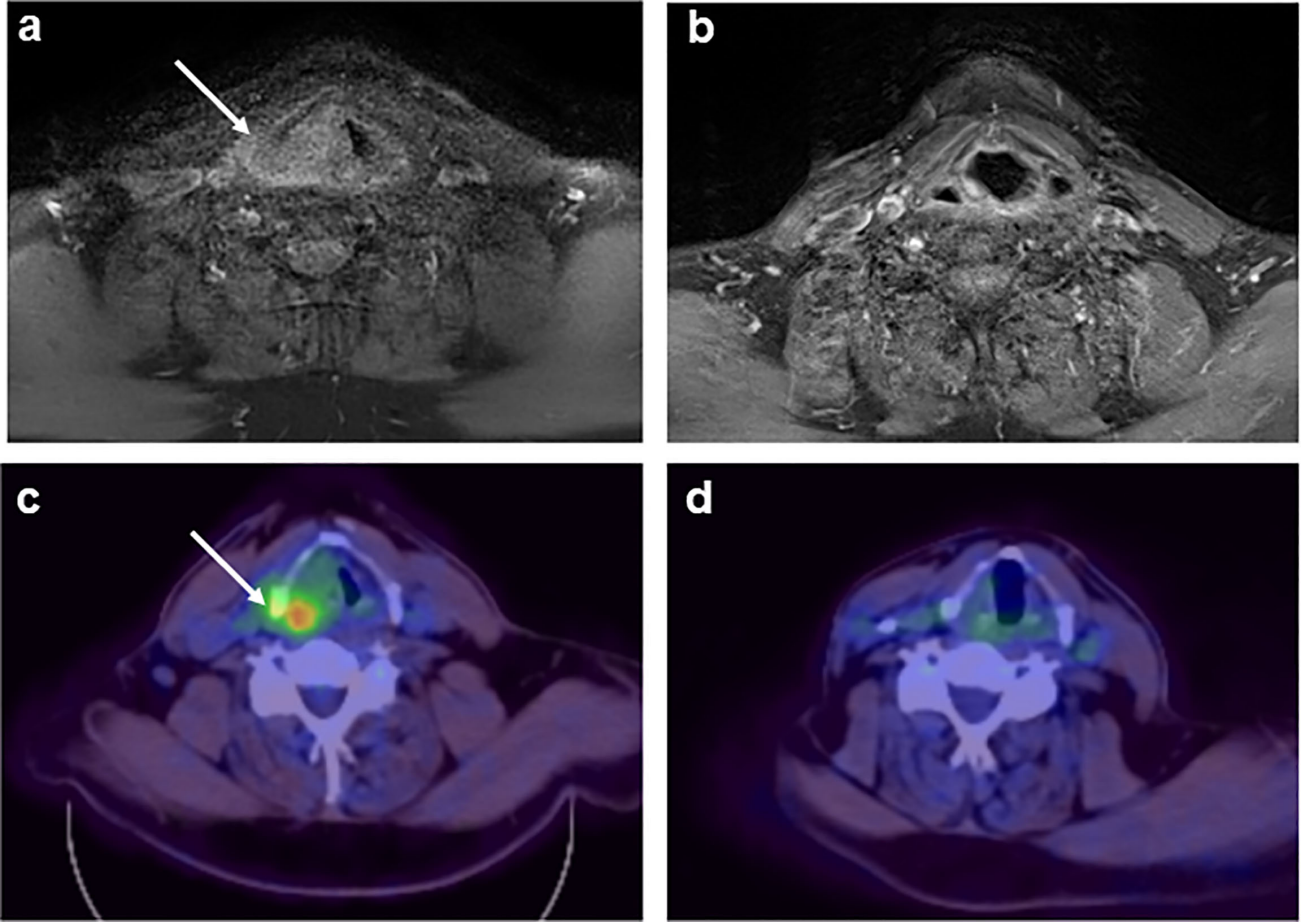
MRI (A, B) and 18-FDG-PET (C, D) of the neck displaying a laryngeal tumor mass at initial diagnosis [(A, C); arrow] and complete remission of the tumor formation after induction + consolidation I (B, D).
